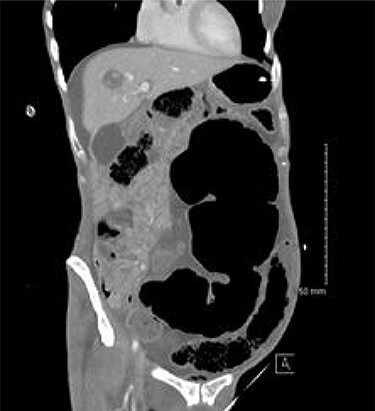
CT appearance suggestive of SV with collapsed right colon and no appreciable CV.
